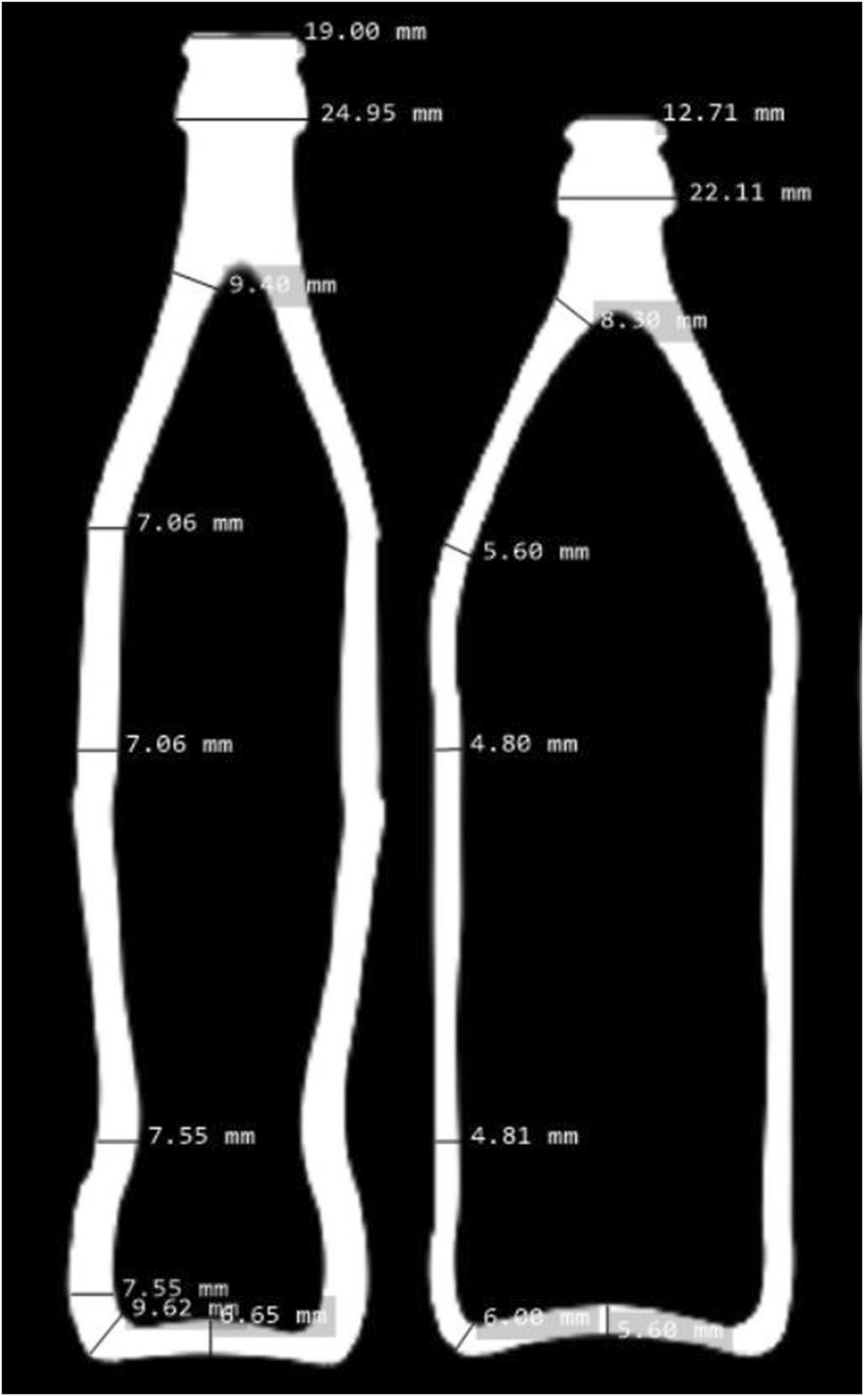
Axial slices of a CT scan of the used 0.5-l and 0.33-l glass bottles
